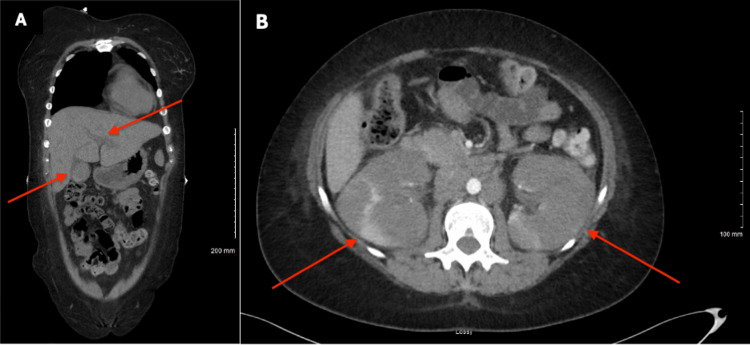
CT abdomen and pelvis without contrast demonstrating hepatobiliary and renal abnormalities (A) Coronal view showing intrahepatic ductal dilatation and increased density within the gallbladder lumen, suggestive of biliary sludge or gallbladder debris (red arrows). (B) Axial view demonstrating bilateral renal enlargement (red arrows). Imaging also revealed increased retroperitoneal soft tissue fullness compared with prior studies, consistent with progression of a known lymphoproliferative process.
